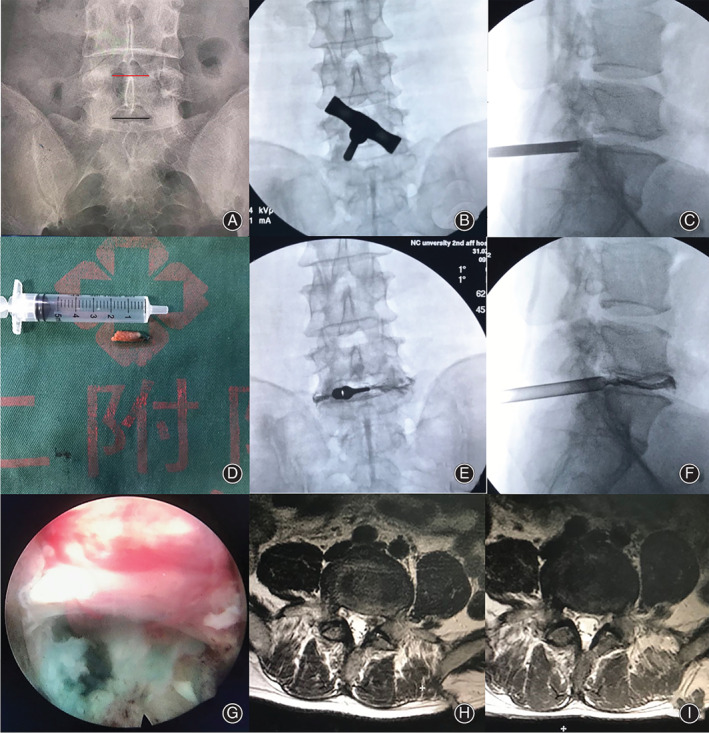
Typical case two. (A) Lumbar anterior–posterior X‐ray image. Narrow interlaminar window of L_5_S_1_ level. (B and C) The trephine was used for laminoplasty at the safety zone. (D) Resected bone from the safety zone. (E and F) Working cannula was inserted at the safety zone. (G) The S_1_ nerve root was completely released. (J and K) Preoperative and postoperative MRI showed good decompression of the left S_1_ nerve root.
